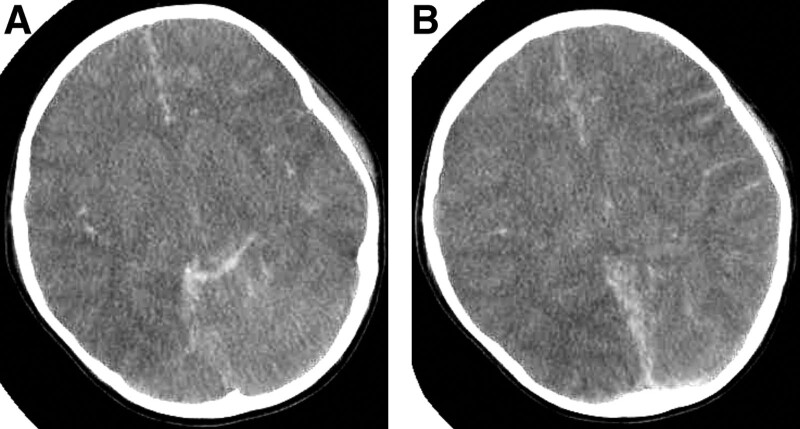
Head CT: The midline structure of the brain is centered, the whole brain parenchyma is swollen, the supratentorial ventricular compression disappears, the density of the brain parenchyma is low, the gray white matter boundary is unclear, and the midline, bilateral cerebral sulci, and tentorial margin of the cerebellum have linear density increases, and the medullary structure is not clearly displayed. No obvious bone abnormality is found in the skull. The whole brain swelling may change with a small amount of subarachnoid hemorrhage, and cerebral hernia may occur (A, B). CT = computed tomography.
